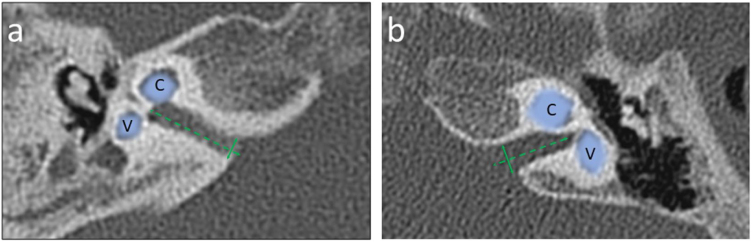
Exemplary measurements of the Internal Auditory Canal (IAC) diameter in an axial section of a computed tomography made at the internal acoustic opening (*porus acusticus internus)*, the IAC diameter (solid line) was determined perpendicular to the longitudinal axis (dashed line) of the IAC; Panel a – narrowed IAC with diameter 2.70 mm (case #2); Panel b ‒ normal sized IAC with diameter 3.76 mm (case #1). Cochlea (C) and Vestibule (V) are marked with a line art over for easier identification.
